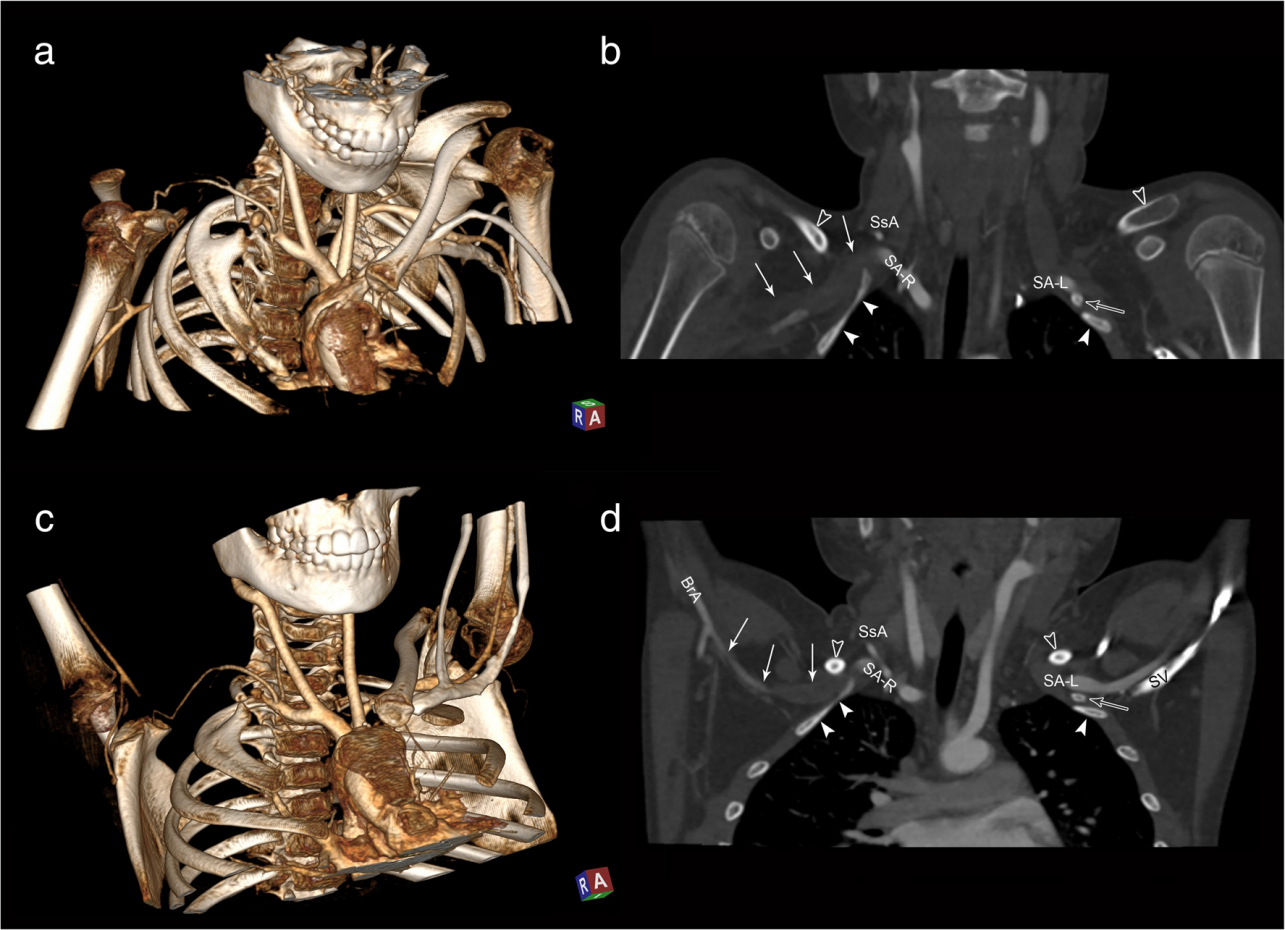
Contrast-enhanced CT scans at two arm positions (a, b—anatomical position; c, d—overhead abduction). Right clavicle removed from 3D reconstructions (a, c) for visualization of cervical-1st rib complex. Coronal cuts (b, d) show subclavian arteries (right, SA-R; left, SA-L) passing between the clavicle (empty arrowhead) and first rib (white arrowheads). Occlusion within right SA (white arrows) reaches the right brachial artery (BrA), supplied by right suprascapular artery (SsA). Contrast medium visible on the right within the proximal SA and SsA. Empty arrow shows left cervical rib. Note the distance between the clavicle and 1st rib on both sides
